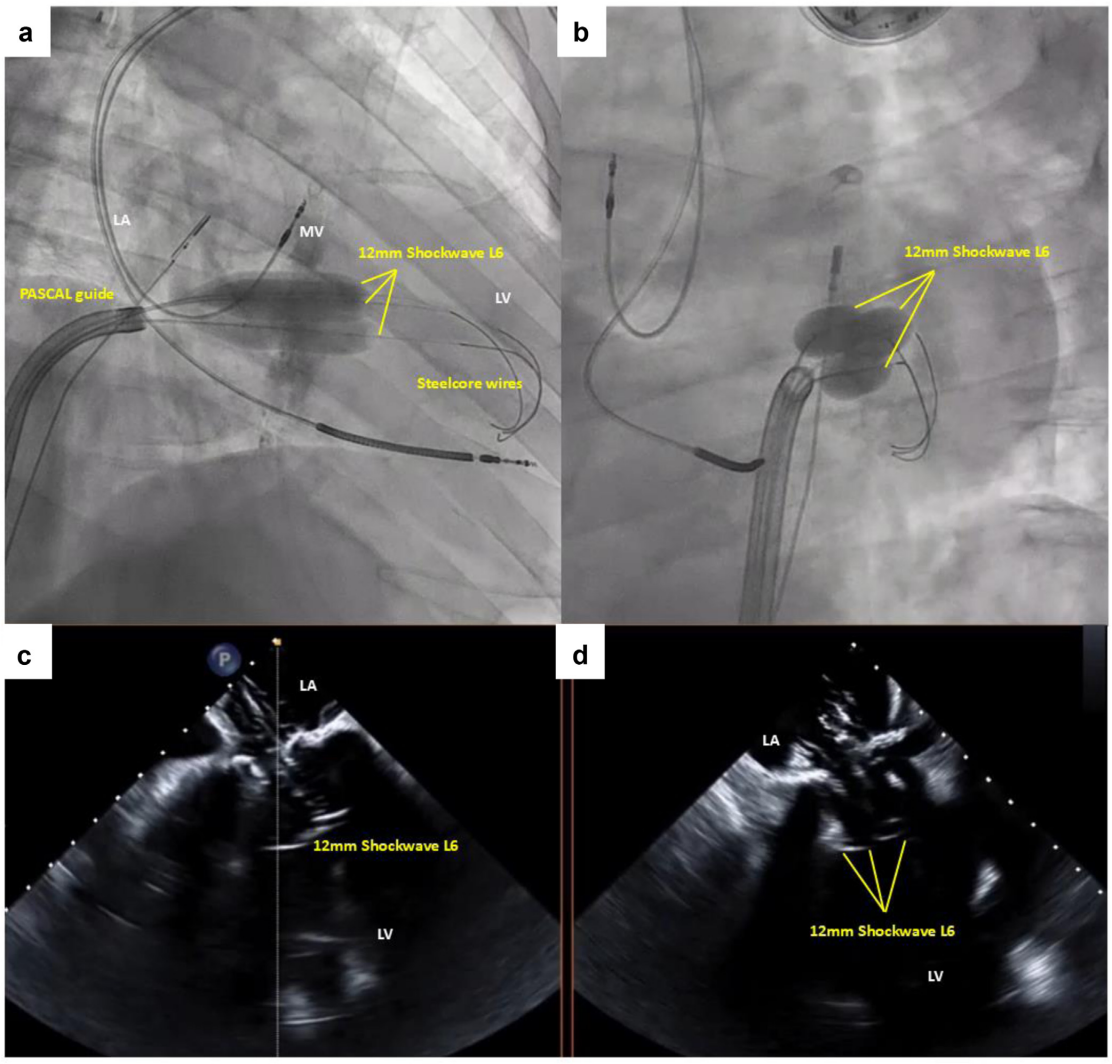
The MATRIX procedure. (a, b) Fluoroscopic images demonstrating full annular apposition with 3 × 12 mm shockwave balloons inflated over 3 guidewires. (c, d) Transesophageal echocardiographic images demonstrating full annular apposition with 3 × 12 mm shockwave balloons. Abbreviations: LA, left atrium; LV, left ventricle; MATRIX, Mitral Annulo-TRIpsy in eXtreme risk patients; MV, mitral valve.
